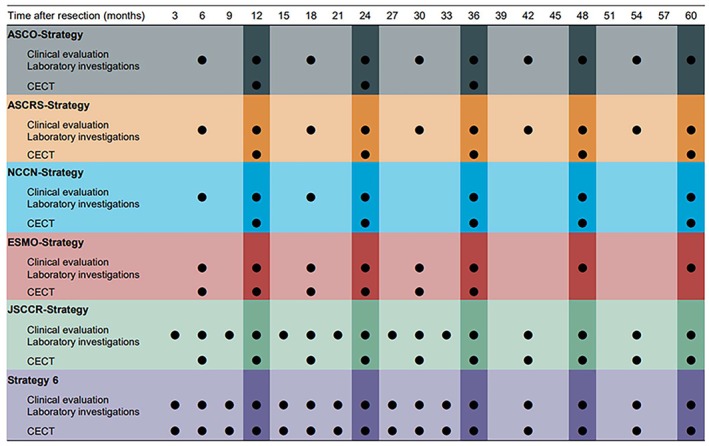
Postoperative surveillance programs evaluated in this study. The timing of the examinations in each surveillance program is shown. CECT, contrast‐enhanced computed tomography.
